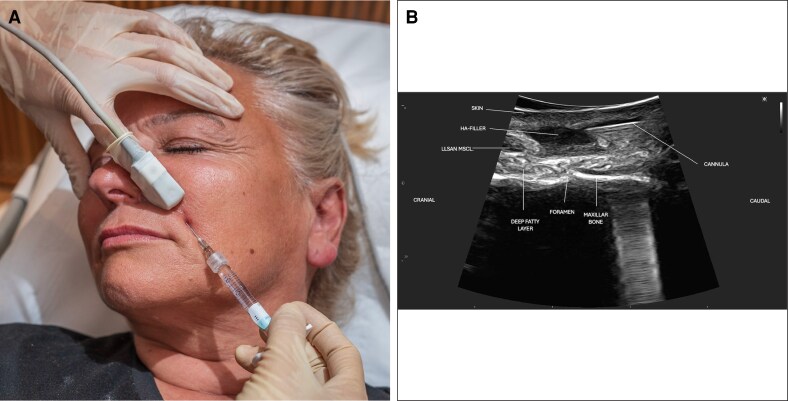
In a 59-year-old female patient, (A) clinical view shows ultrasound-guided hyaluronic acid injection into the nasolabial fold using the SIBUS-In probe. (B) The corresponding grayscale ultrasound image demonstrates the hyaluronic acid filler as hypoechoic material along the tip of a 25 G cannula, fully visualized with the in-plane technique.
